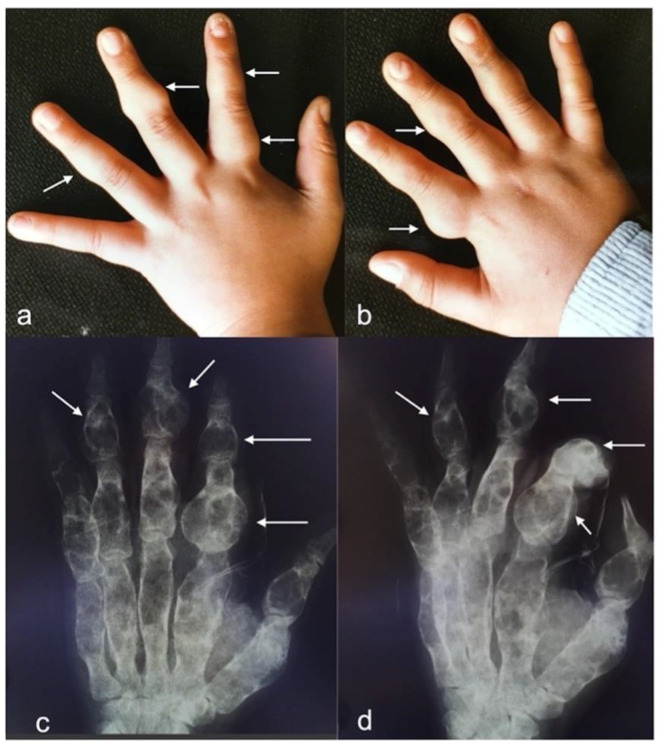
Photograph of hands. (a,b) Left and right hands with multiple palpable bony nodules (white arrows). (c,d) X-rays of left hand showing multiple enchondromas at the proximal phalanx of the second finger and intermediate phalanxes of second, third, and fourth fingers.
